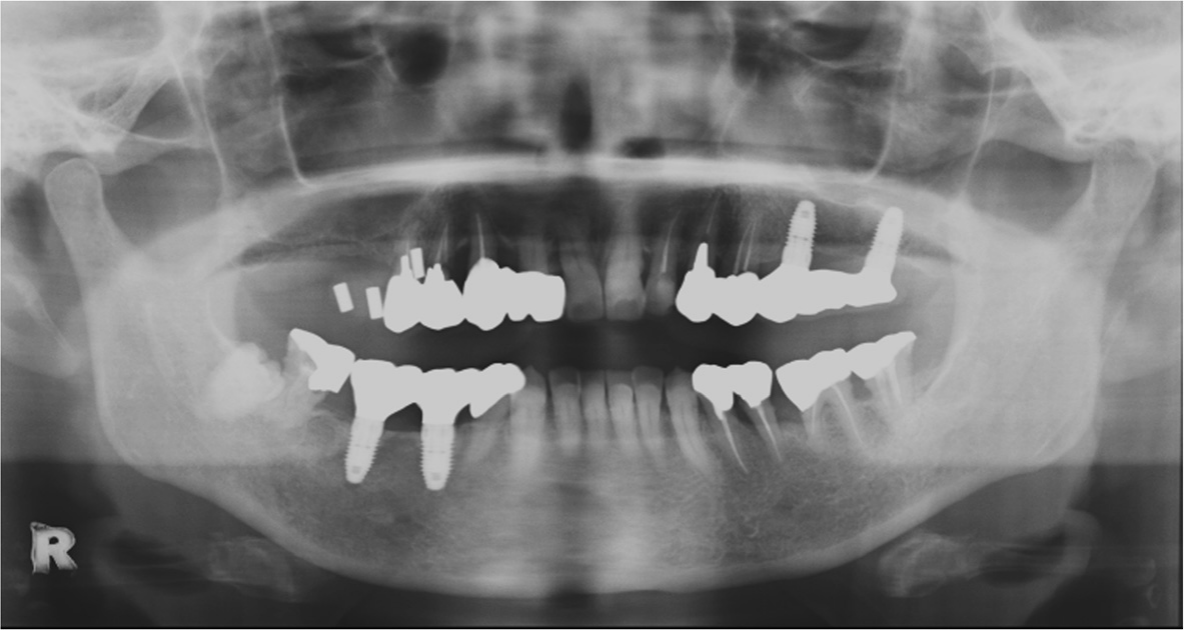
Preoperative panoramic radiograph finding
